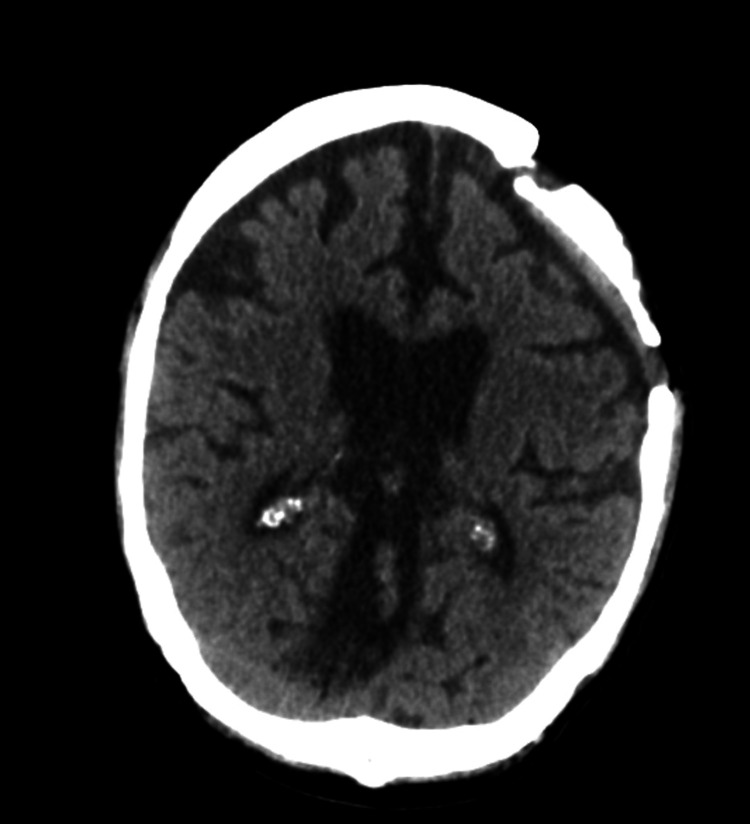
CT of the brain without contrast, initial, showing severe osteomyelitis of the left frontoparietal bone.
